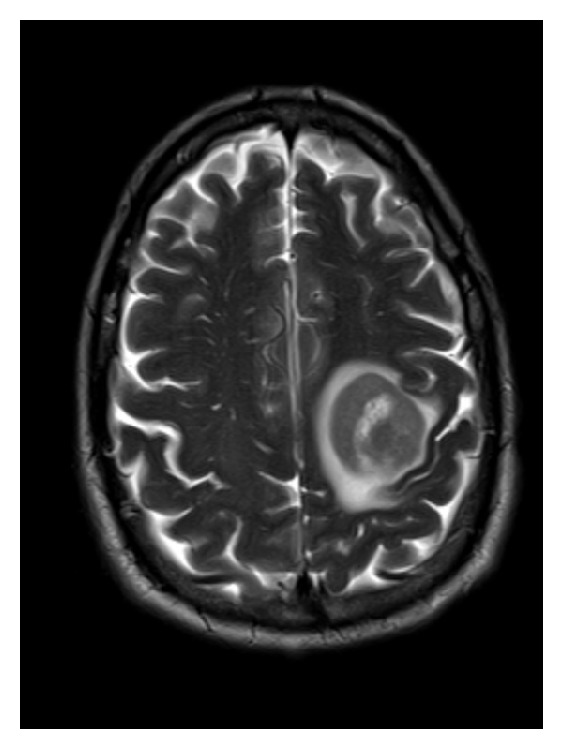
MRI of the brain showed a large mass in the left frontal area T2/FLAIR. Mild surrounding vasogenic edema and mass effect.
